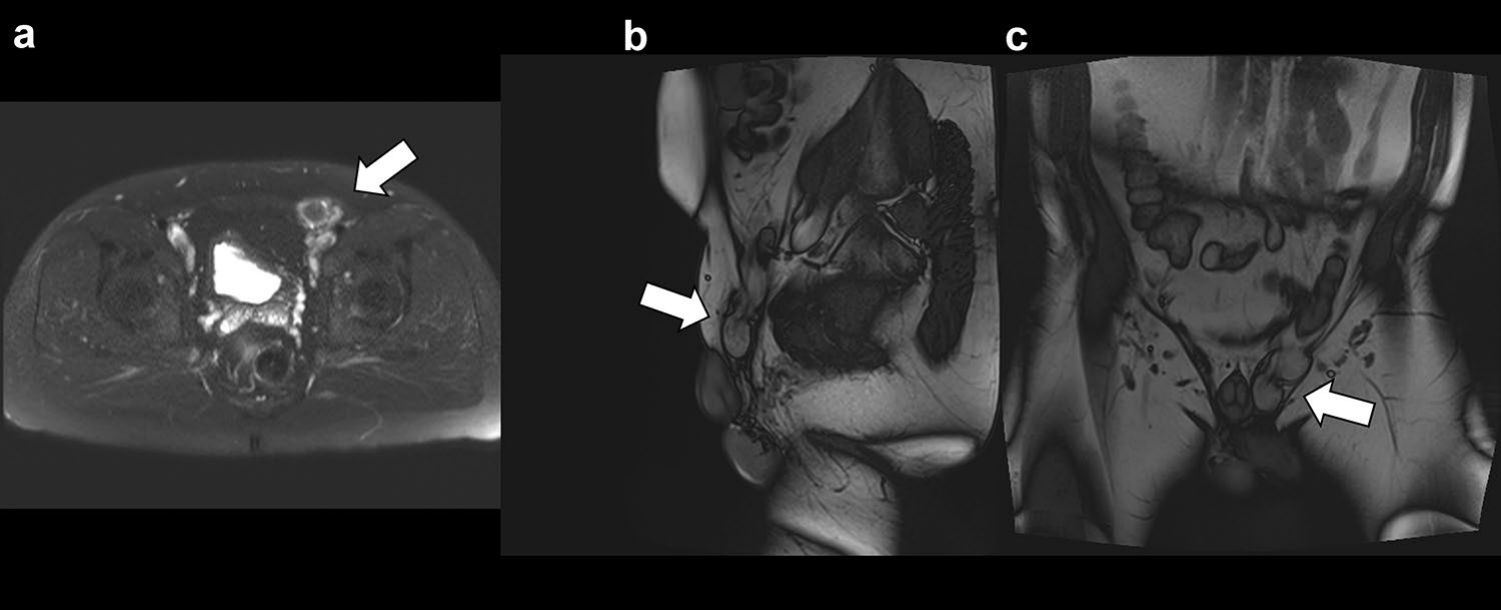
Case of a 32-year-old patient suffering from pain in the left groin for 1 day. Physical examination showed no certain sign of inguinal herniation. Ultrasound showed an uncertain formation in the left groin. For further investigation, a dynamic MRI examination was performed. Standard static MRI examination with axial T2-Haste sequence a and dynamic MRI examination with sagittal b and coronar c T2w. Fast imaging with steady precession (TRUFI) confirmed a herniation in the left groin
